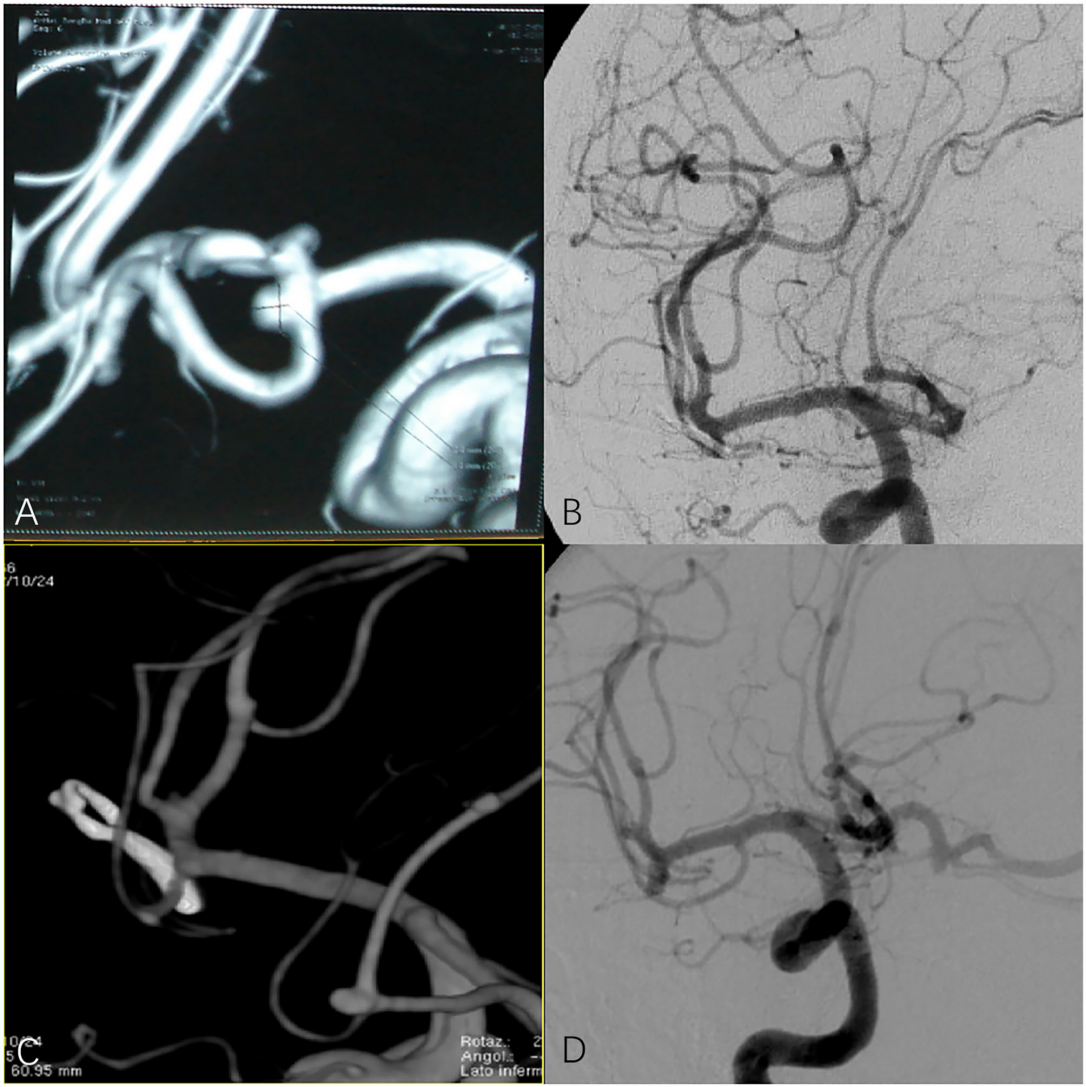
(A,B) Preoperative digital subtraction angiography (DSA) or computed tomography angiography (CTA) in patients with anterior communicating artery aneurysm. (C,D) CTA and DSA of patients with anterior communicating artery aneurysm after surgery.
